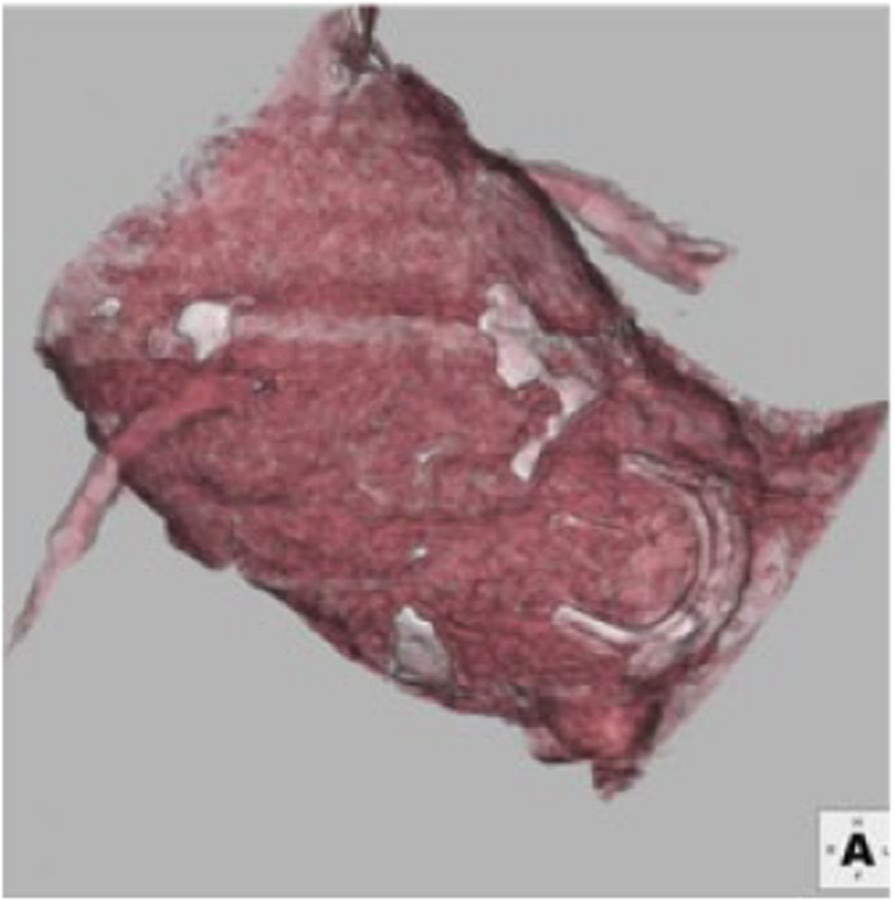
The 3D computed tomography image showed dislodgement of the bioprosthesis entirely approximatley 20 mm beneath the aortic annulus into the left ventricle outflow tract
